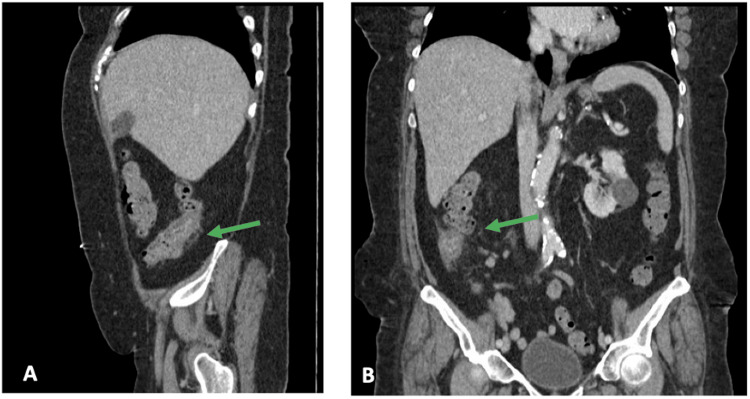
Computed tomography of the abdomen and pelvis (A) Sagittal and (B) coronal views, taken on the day of presentation at the Ronald Reagan UCLA emergency department, show mid-ascending wall thickening spanning approximately 4 cm with trace surrounding stranding (green arrow).
